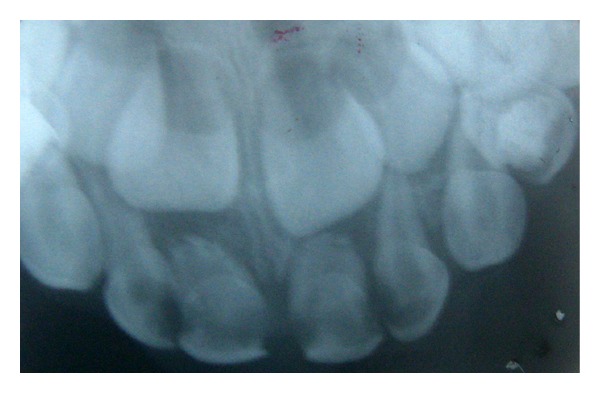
Radiographic image presenting periapical lesion and increased pericementary space.
